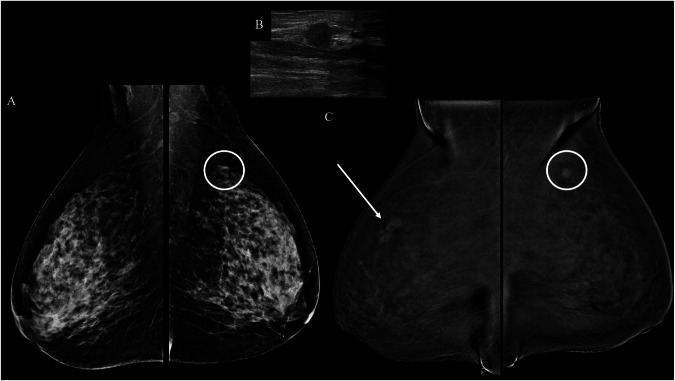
Preoperative CEM in a 61-year-old woman with dense breasts. An invasive carcinoma of the upper outer quadrant of the left breast was previously diagnosed by ultrasound-guided biopsy. LE MLO images (A) show an irregular mass in the left upper outer quadrant (white circle), with corresponding ultrasound findings shown in (B). Recombined MLO images (C) reveal a mass in the site of the index lesion in the upper-outer left quadrant and an additional area of heterogeneous non-mass enhancement in the upper-central region of the right breast (white arrow). The latter was negative at US-second look and showed a subtle architectural distortion on digital breast tomosynthesis (DBT), which resulted in DCIS at DBT-guided biopsy. Final pathology after bilateral surgical excision confirmed a 20-mm grade 3 DCIS in the right breast and an 8-mm grade 3 invasive papillary carcinoma (Luminal B) in the left breast
